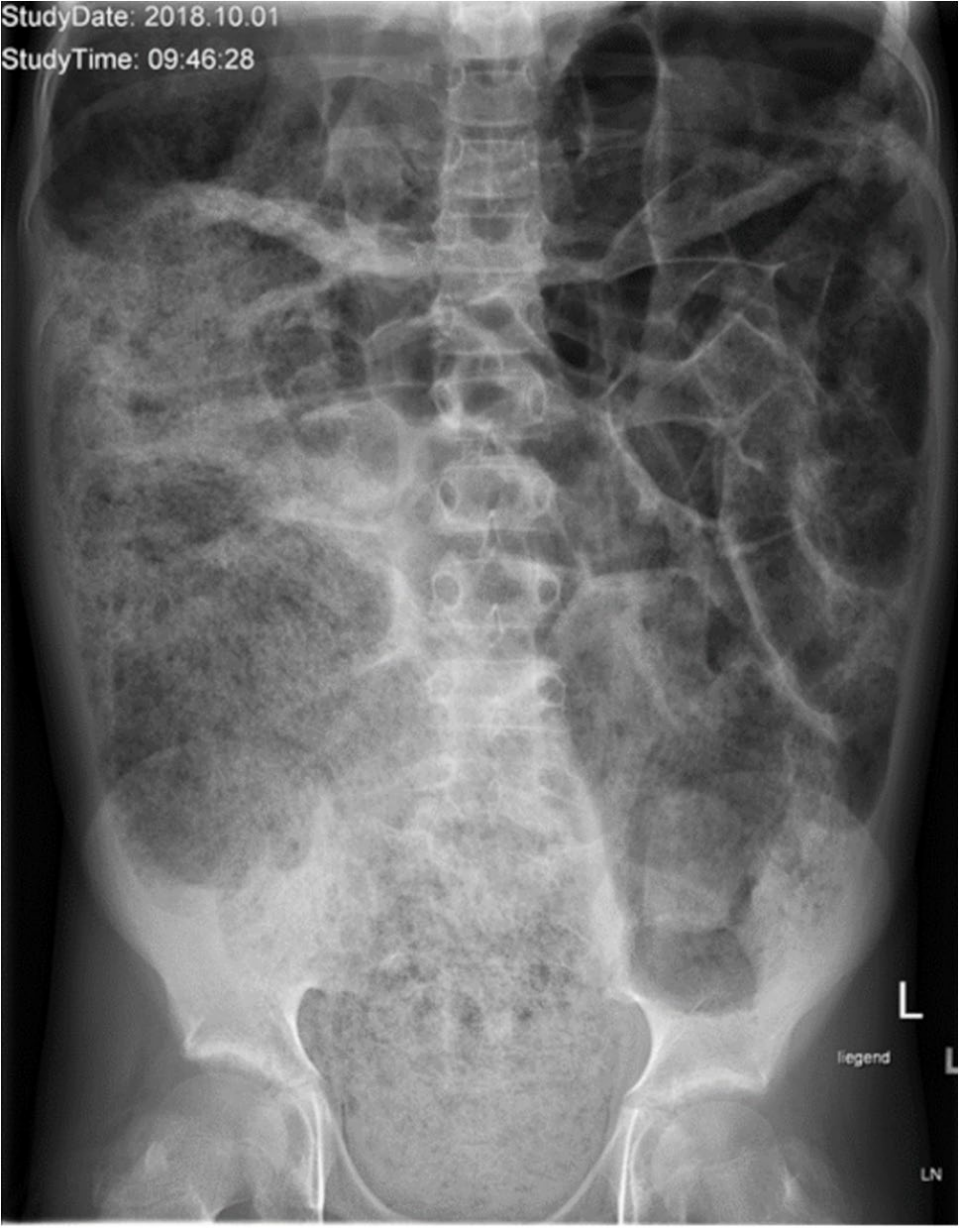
An anteroposterior abdominal radiograph in a 10-year-old boy with constipation (the same patient as in Fig. 8) shows massive fecal overloading and small bowel dilatation. The Barr score is 21 points—well above the normal limit of 10 points
